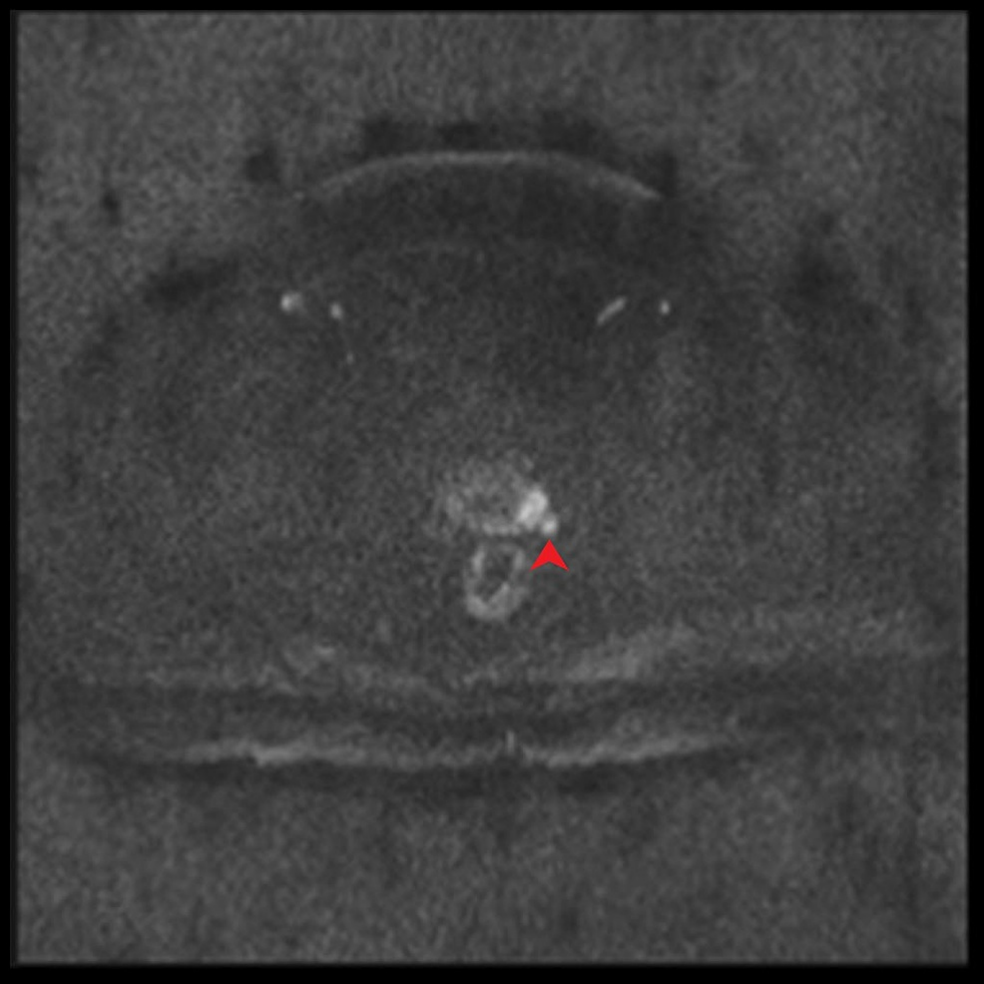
DWI sequence showing prostatic carcinoma with an extracapsular extension on the left side (arrowhead). DWI: diffusion-weighted imaging.
